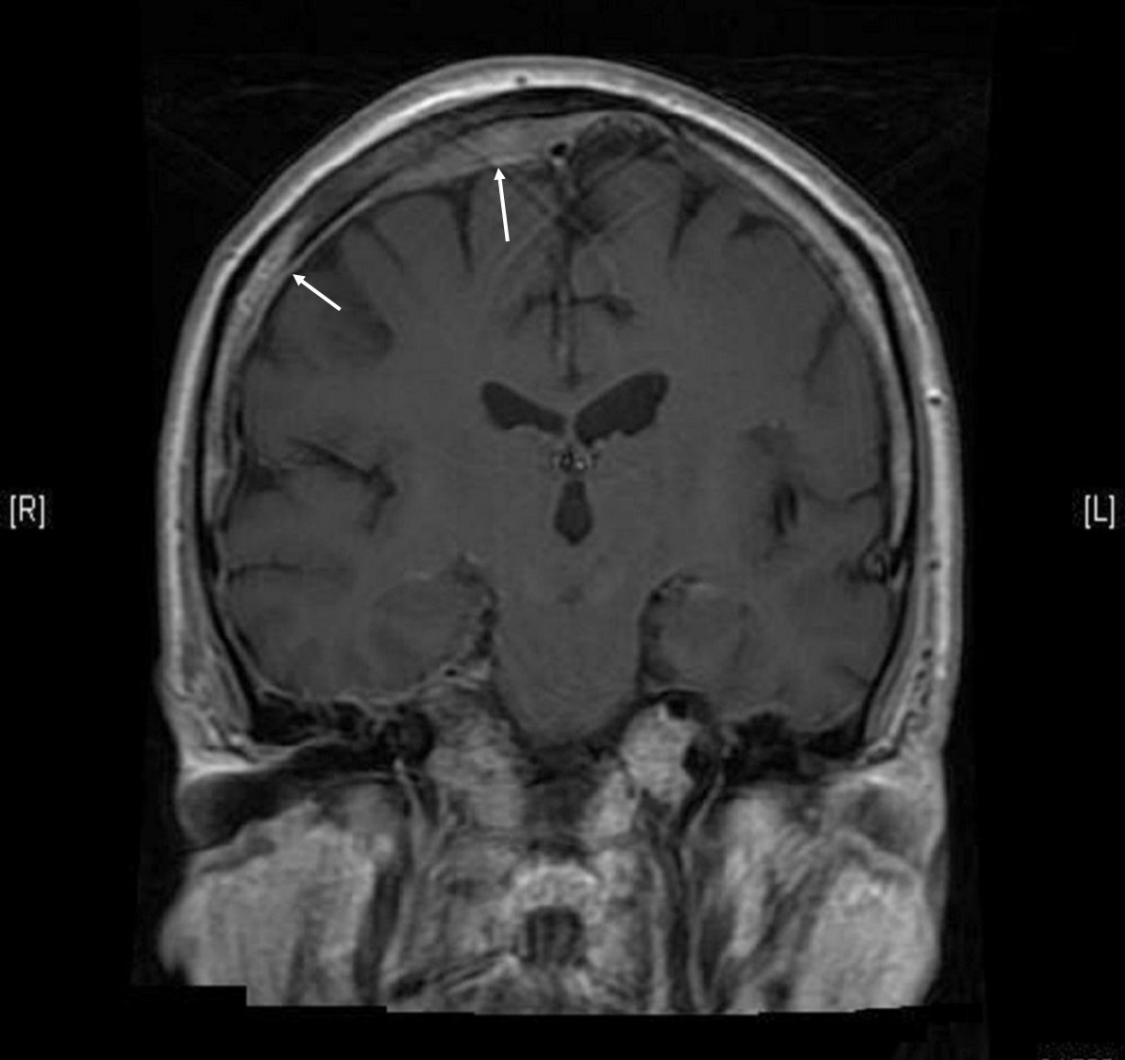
MRI brain Extensive smooth dural involvement over the cerebral hemispheres more prominent on the right. MRI: Magnetic resonance imaging
